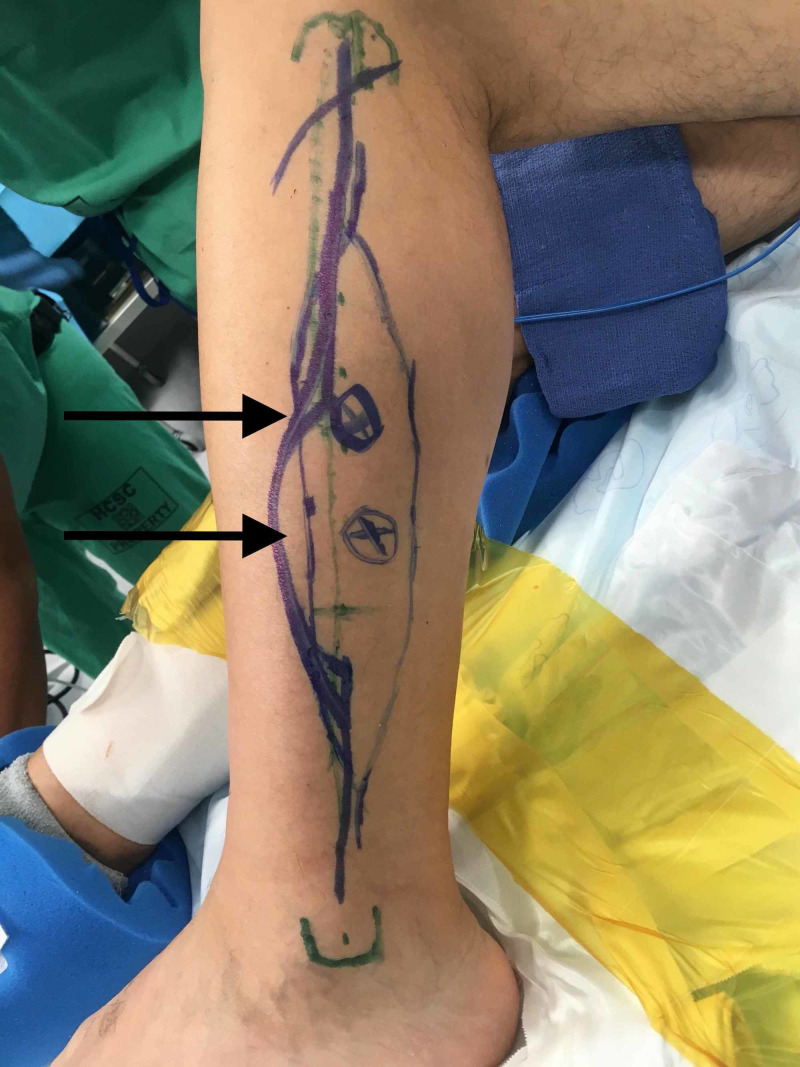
Preoperative fibula flap markings including dopplerable perforators (arrows)
